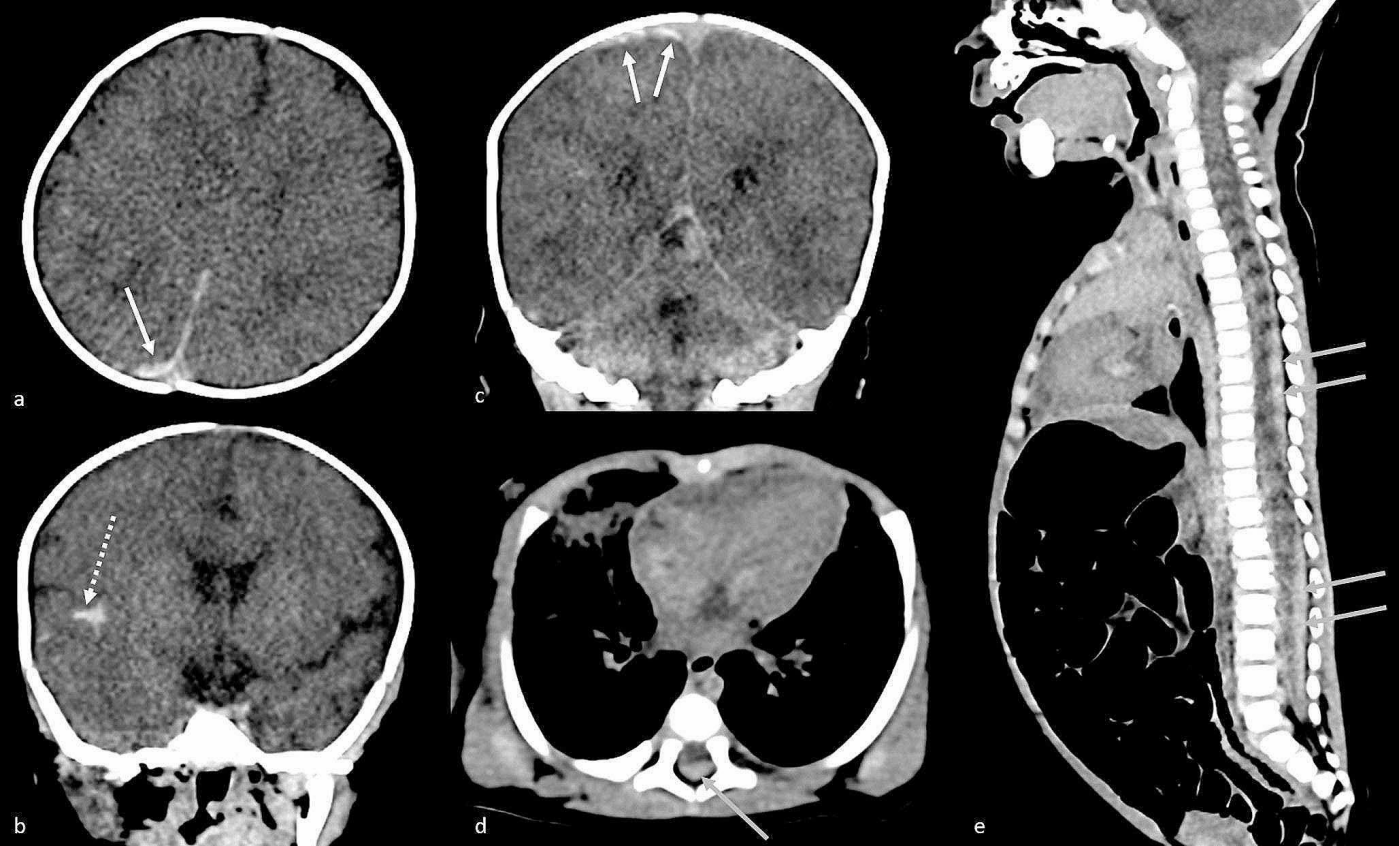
Post mortem computed tomography. Soft tissue reconstruction. On the axial view of the brain (a), subdural hematoma is visible as a hyperdensity of the subdural space of the parietal convexity (arrow). On the coronal view of the brain (b, c), subarachnoid haemorrhage is visible as a spontaneous hyperdensity of the sylvian fissure (dotted arrow) and temporal subdural hematoma is visible as a lens-like hyperdensity of the subdural space (arrowhead). On the axial (d) and sagittal view (e) of the spinal cord, epidural hematoma is visible as a spontaneous epidural hyperdensity around the spinal cord (gray arrows)
